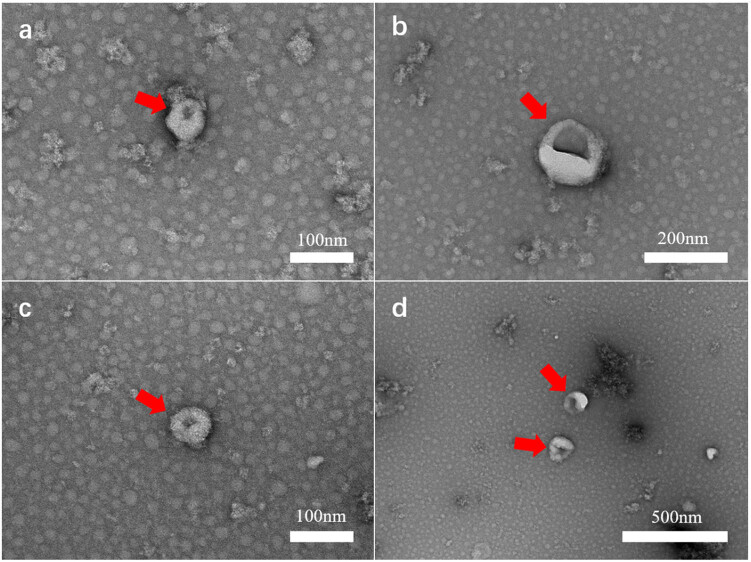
Exosomes under TEM. (a) and (b) Exosomes from BV-treated T cells (BVT-exo). (c) and (d) Exosomes from control T cells (CT-exo). Exosomes are highlighted with red arrows, and the scale bar is marked in the lower right corner of each panel.
